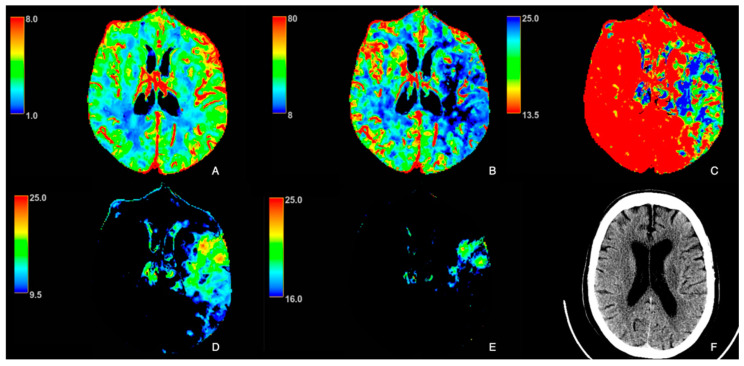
A 83-year-old female patient with acute ischemic stroke due to left middle cerebral artery occlusion under statin therapy. (A): Cerebral blood volume (CBV); (B): cerebral blood flow (CBF); (C): mean transit time (MTT); (D): $T_{max}^{9.5-25s}$; (E): $T_{max}^{16-25s}$; (F): axial CT brain. NIHSS onset: 5.
